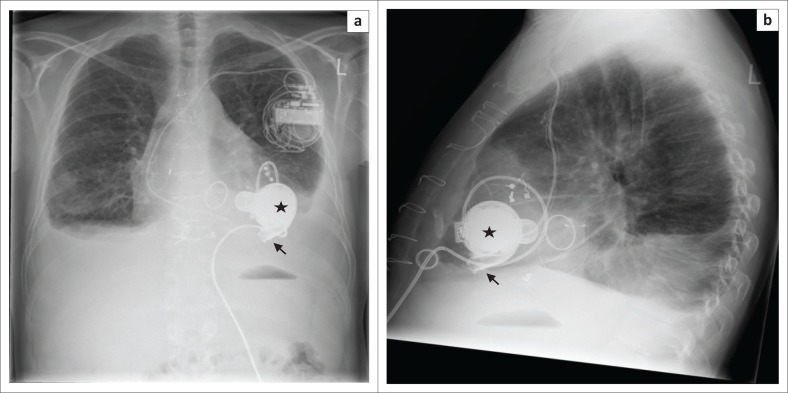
Frontal (a) and lateral (b) chest radiographs showing a HeartWare left ventricular assist device in a 41-year-old male with familial dilated cardiomyopathy. Note that its outflow cannula (arrow) is without angulation and it has a circular impeller unit (star).
